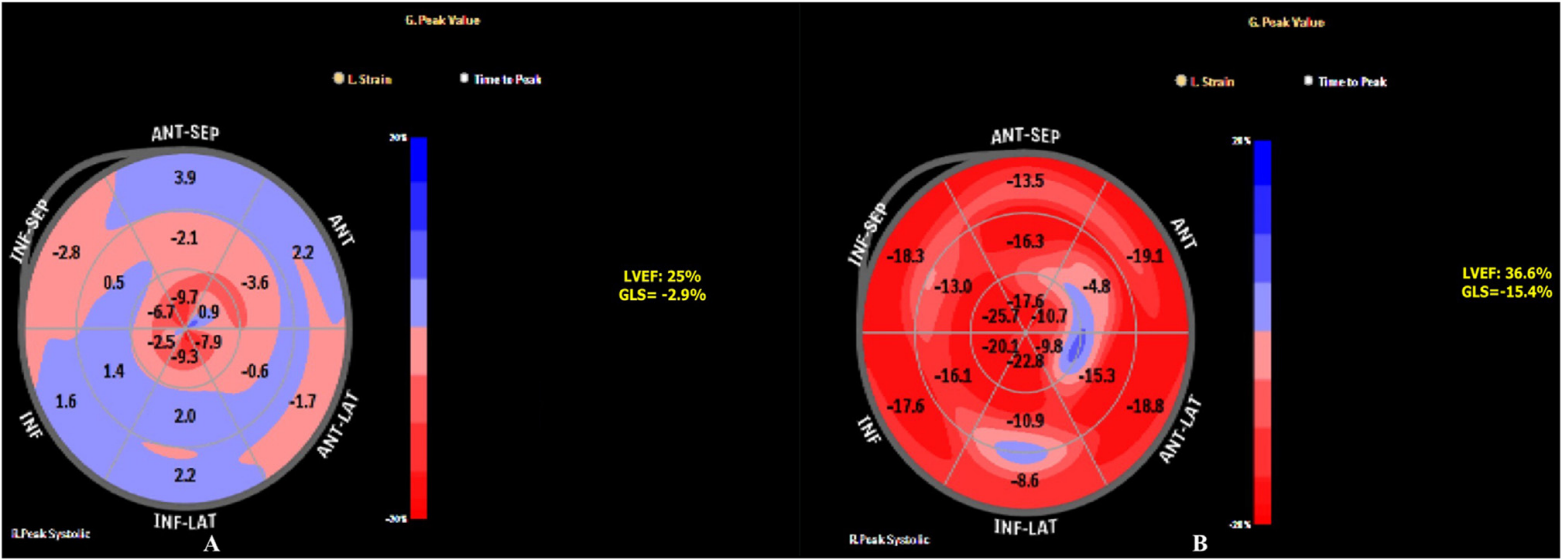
A Pre-intervention speckle tracking echocardiography bull's eye plot of the same patient as in Fig. 1A and B showing global longitudinal strain to be −2.9% and left ventricular ejection fraction of 25%. Fig. 2B: Post-intervention speckle tracking echocardiography bull's eye plot at 6 months of follow-up showing improvement in left ventricular global longitudinal strain to −15.4% and left ventricular ejection fraction of 36.6%.
